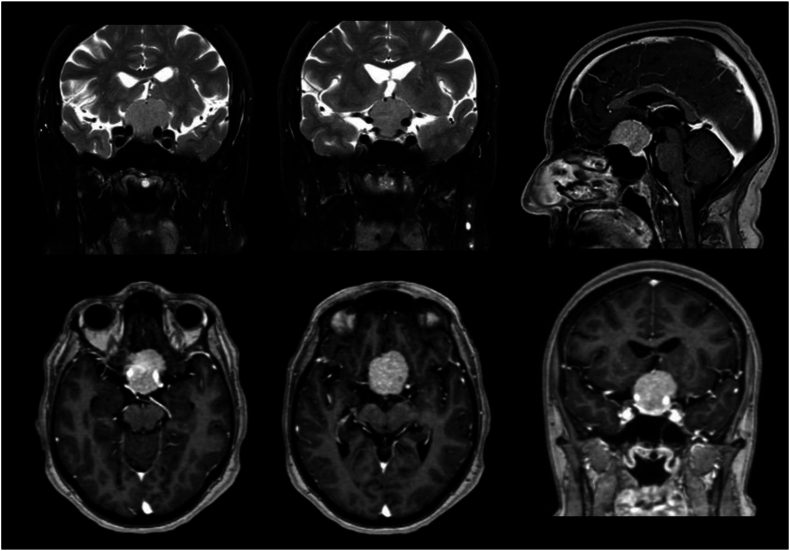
Pre-operative T1-Gd- and T2-weighted imaging showing a tuberculum sellae meningioma. These DICOM data were used to segment and create the 3D-models used for VR and 3D printing.
